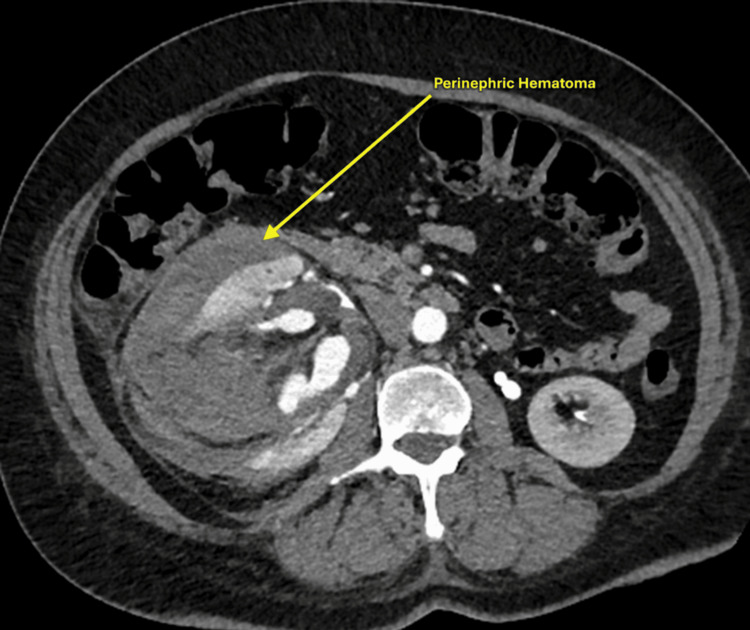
Axial-enhanced CT images demonstrating a large, hyperdense perinephric hematoma (yellow arrow) encasing and compressing the right kidney The hematoma caused significant enlargement of the right renal fossa.
